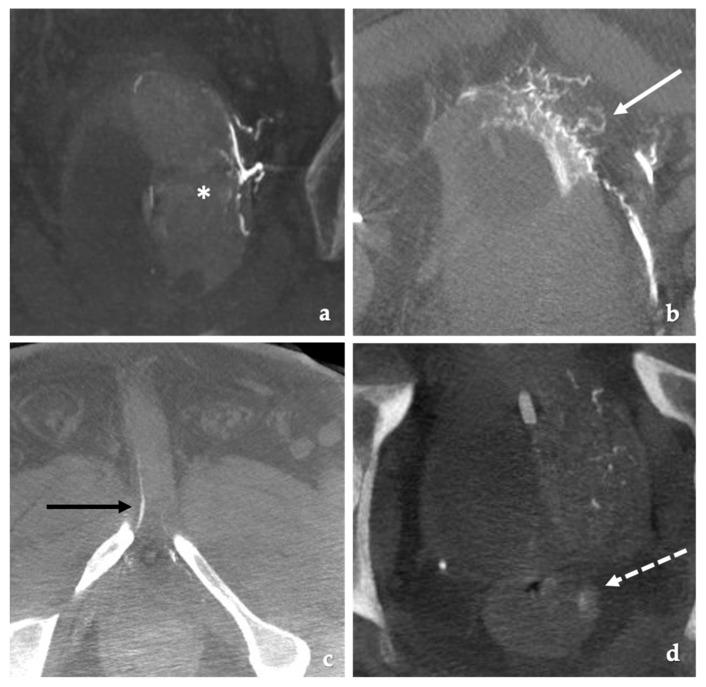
Examples of cone beam CT demonstrating target and non-target embolization. (a) Coronal reconstruction from a cone beam CT acquisition. Appropriate contrast enhancement of the left hemiprostate (white asterisks) with contrast injection of the left prostatic artery. (b) Axial cone beam CT image. Non-target filling of the bladder (white arrow). Foley catheter balloon in the bladder. (c) Axial cone beam CT image. Non-target filling of the penile artery (black arrow). (d) Axial cone beam image. Non-target enhancement of the rectum (dotted white arrow) with contrast enhancement of the prostate.
